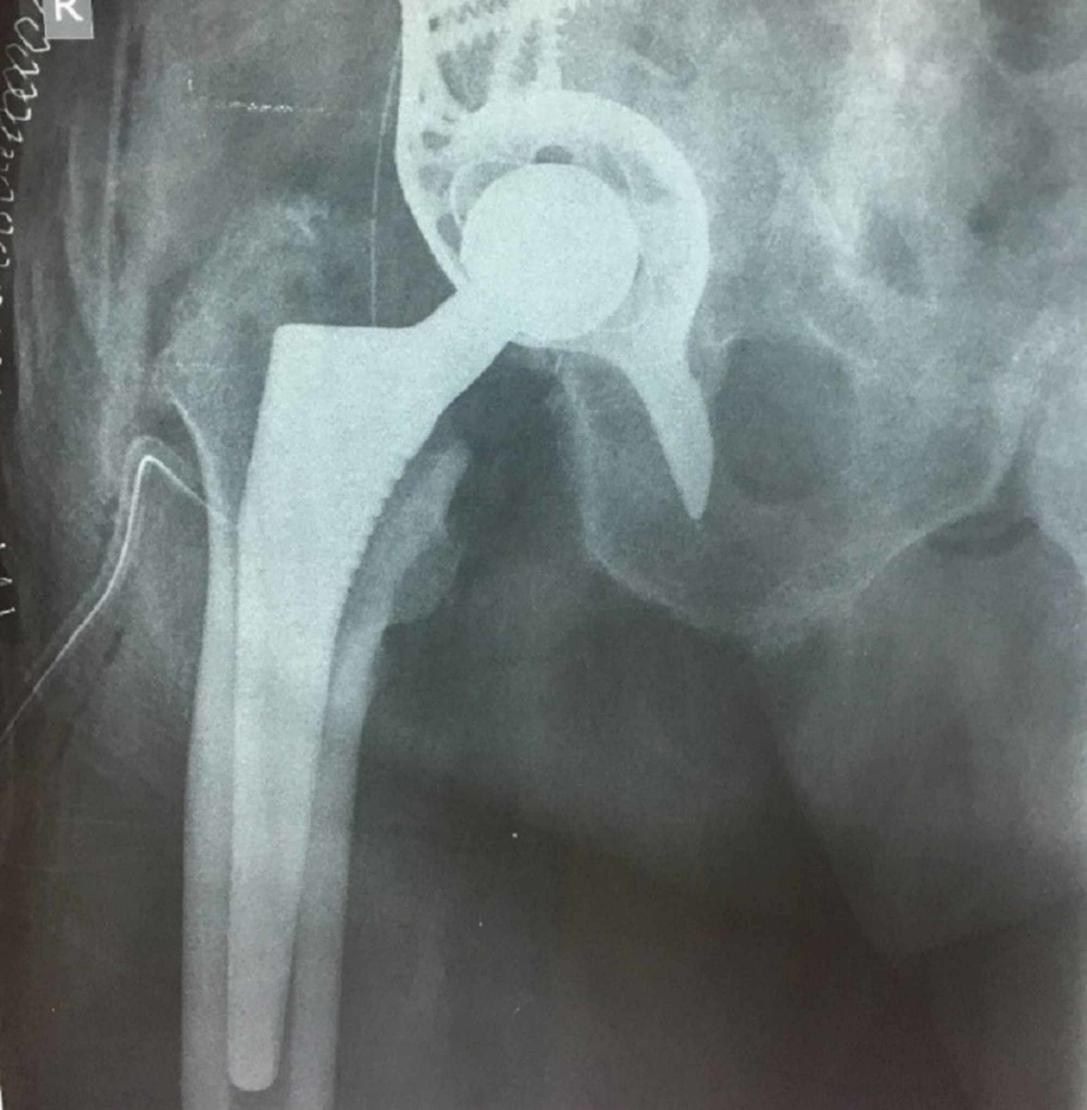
Radiograph of the same patient postoperatively
